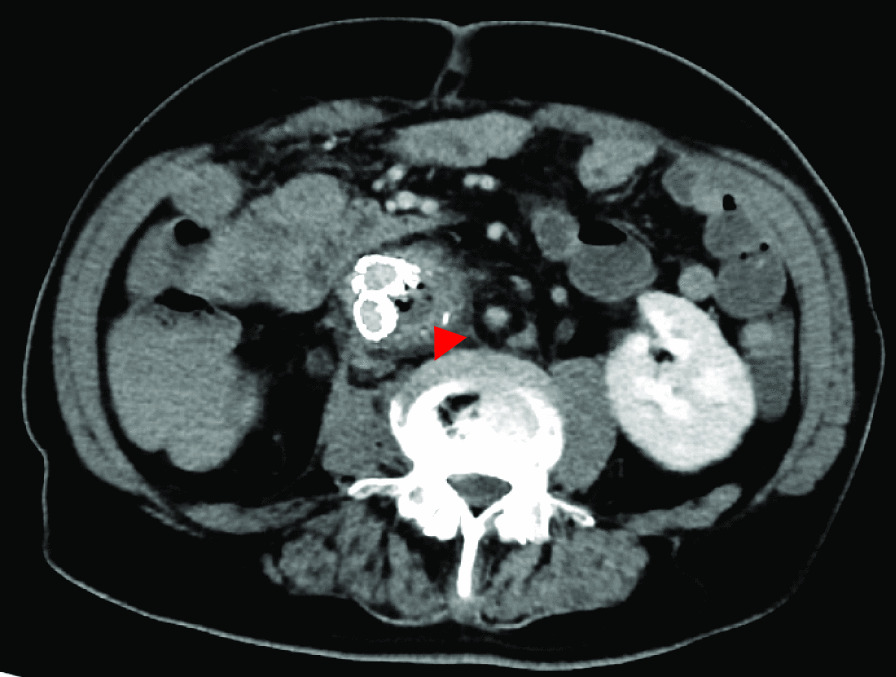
Contrast computed tomography image after upper endoscopy (case 2). Arrowhead: Image of air in the aorta
